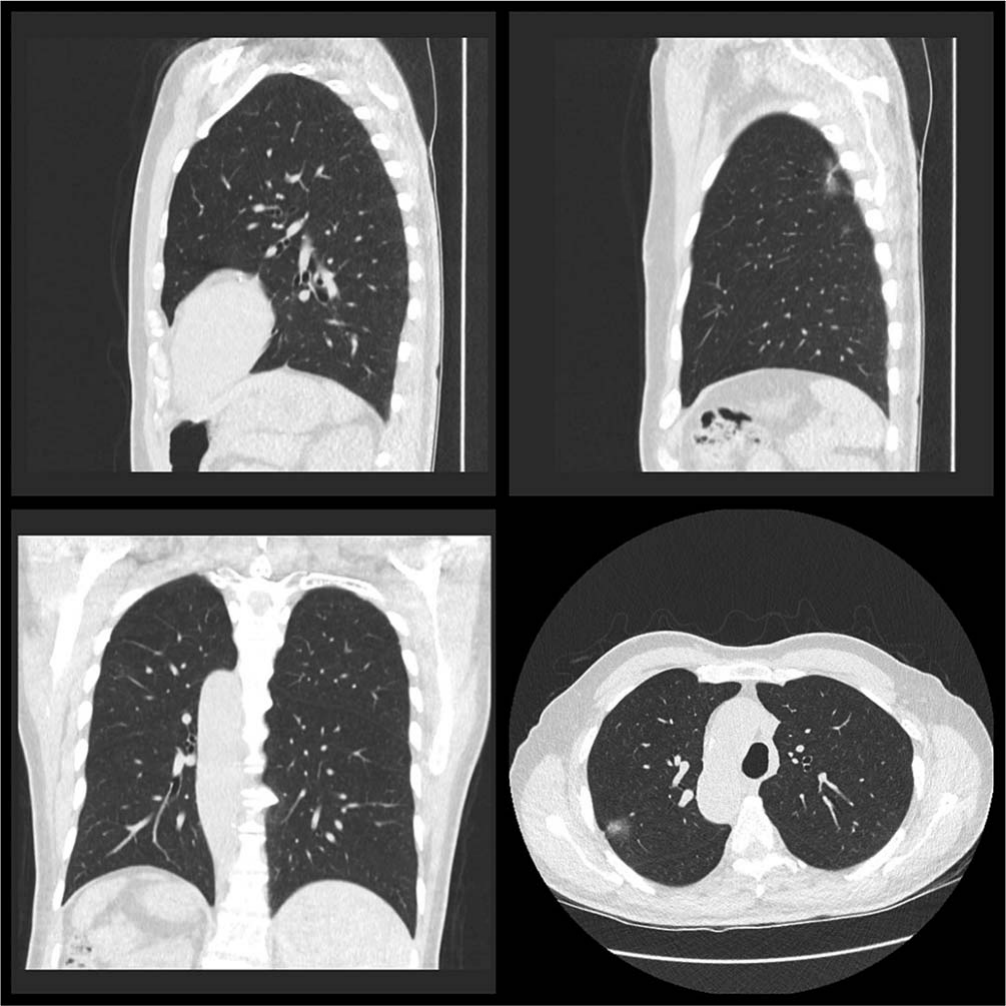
Pre-operative CT thorax, abdomen, pelvis.
